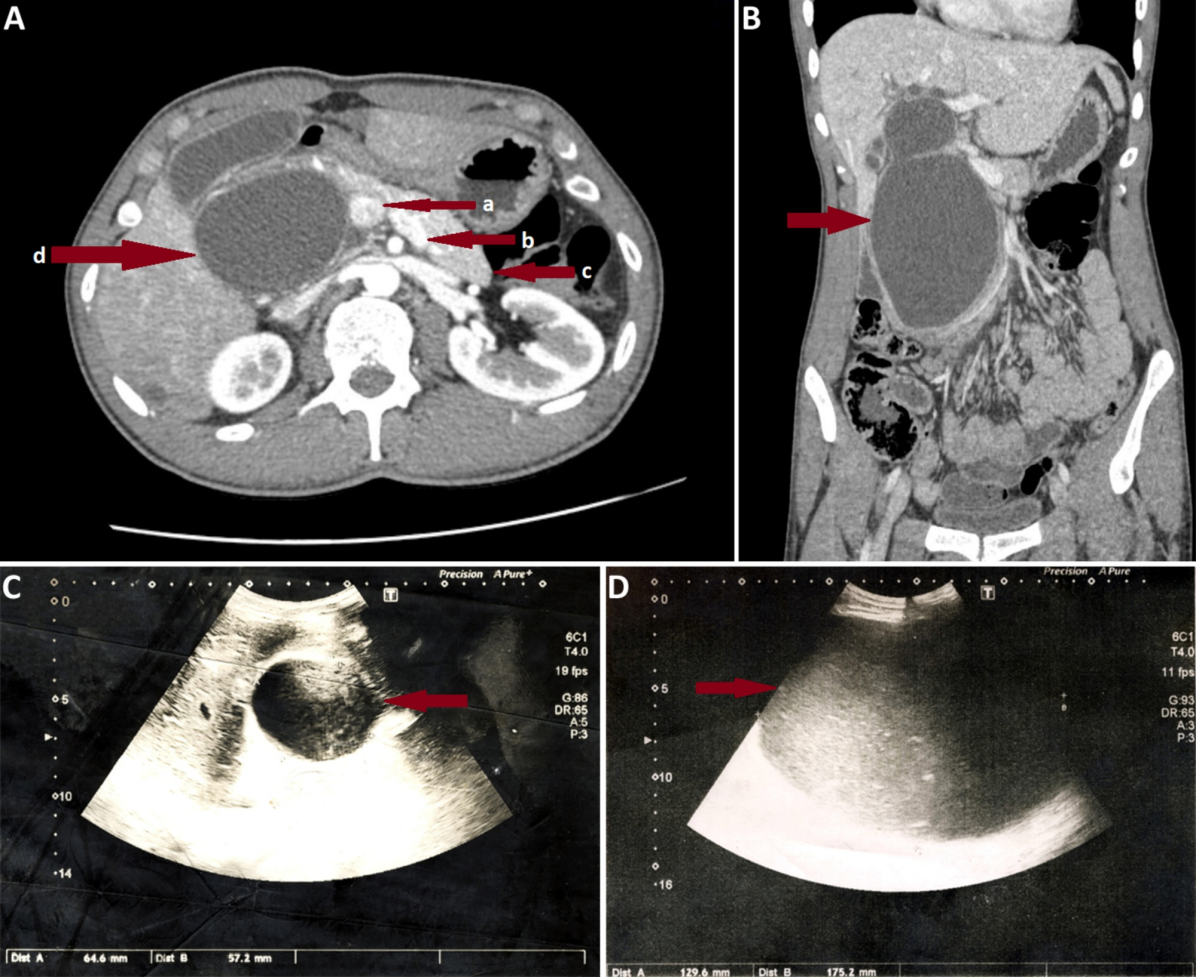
Pictures of the patient's preoperative CT scan and ultrasounds Figures 1A, 1B are axial and coronal images of preoperative CT scan of patient undertaken three weeks prior to admission, which depict the size and extent of the giant Todani type I choledochal cyst. In Figure 1A, a shows portal vein, b shows splenic vein, c shows body and tail of pancreas, and d shows the giant choledochal cyst almost completely replacing the head of the pancreas. In Figure 1B, the arrow shows the choledochal cyst in a coronal image. Figure 1C is an ultrasound image of the patient on the day of admission; the arrow shows the size of choledochal cyst. Figure 1D is an ultrasound image of the patient on the 7th-day post-admission after his condition suddenly deteriorated; the arrow shows the choledochal cyst at this point in time. In Figures 1C, 1D, the sudden increase in size on the 7th post-admission day can be appreciated.
